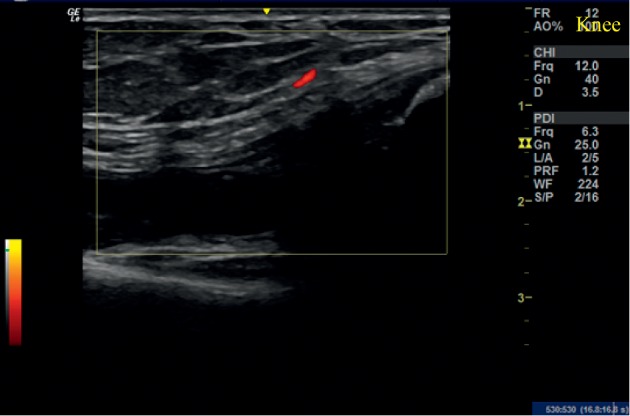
Ultrasound examination of the right knee in case 3.Longitudinal view showing initially synovitis with significant synovial effusion.
